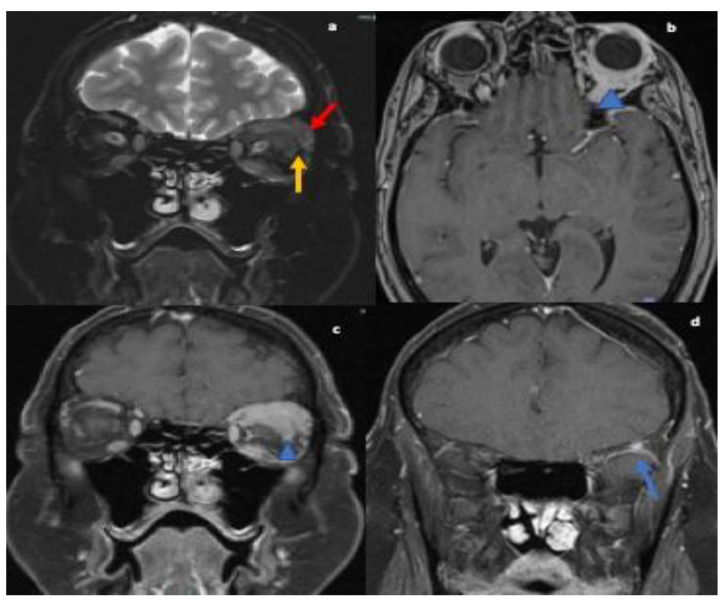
MRI orbit T2 fat-suppressed coronal images reveals nodular thickening of the left lacrimal gland (red arrow; (a)), superior and lateral recti muscles (yellow arrow; (a)). T1-weighted post contrast axial (b) and coronal images (c) showing corresponding enhancement within muscles, lacrimal gland and in intraconal orbits surrounding the globe (blue arrowhead).T1 weighted post contrast coronal image (d) reveals enhancing nodule in the greater wing of left sphenoid concerning for osseous involvement (blue arrow).
